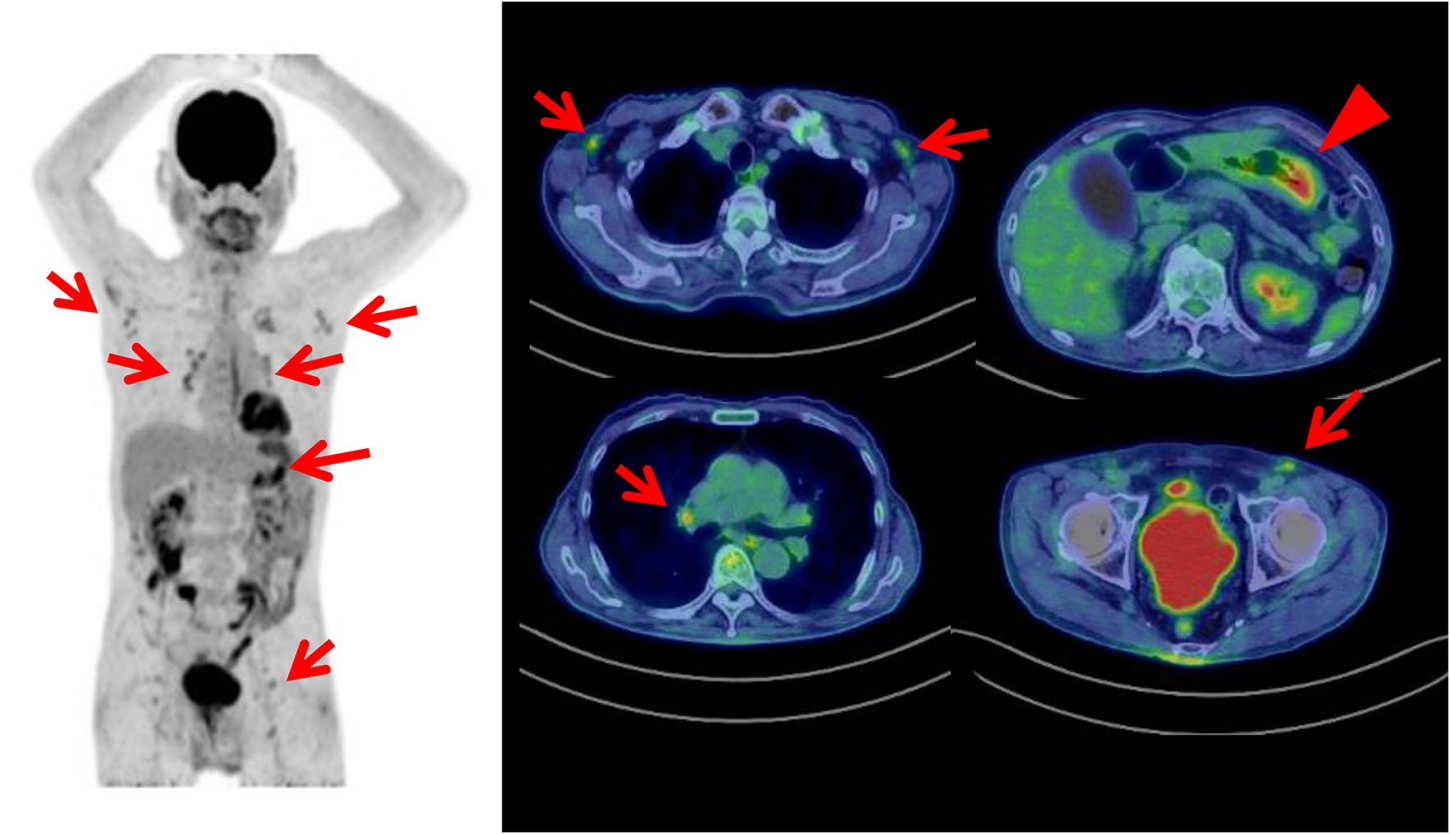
In a 76-year-old male with Sweet syndrome, whole-body FDG PET maximum projection and cross-sectional images showed FDG-avid (SUVmax 2.1) lymphadenopathy in the both axillary and hilar regions and in the both external iliac and left inguinal regions (arrows). The biopsy of the left inguinal lymph node revealed follicular lymphoma. In addition, focal intensive FDG uptake (SUVmax 5.0) was also seen in the lower part of gastric corpus (arrowhead), which was later diagnosed as gastric cancer by endoscopic biopsy
